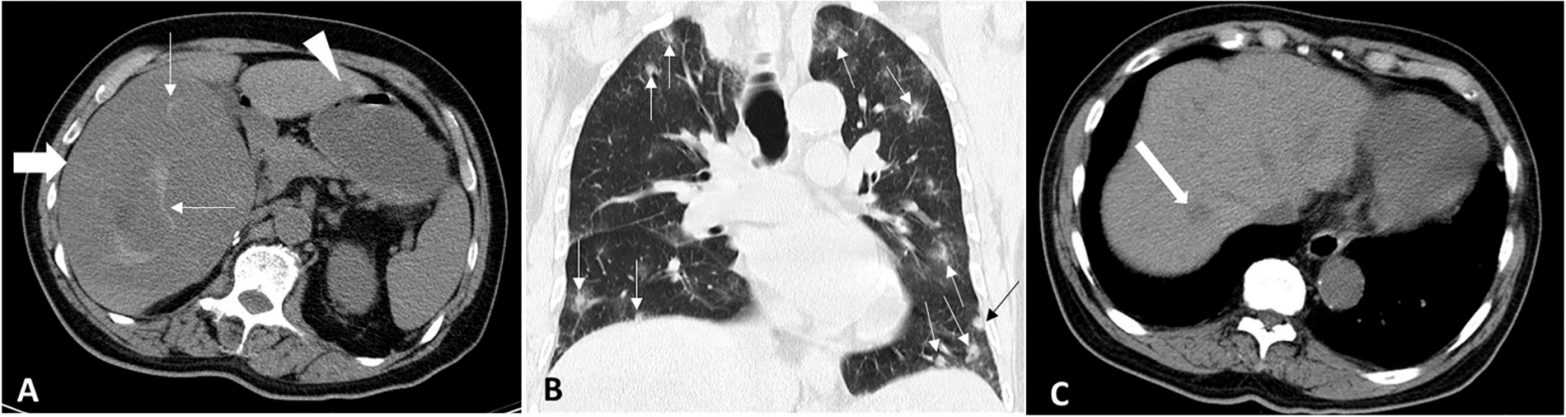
An 82-year-old man with primary hepatic angiosarcoma (case 6). (A) Transverse pre-contrast computed tomography (CT) image shows a dominant low dense mass (thick arrow) in the right lobe with small intra-tumoral high density foci (thin arrows), which suggest hemorrhage. Another smaller tumor in the left lobe of the liver is also noted (arrowhead). (B) Coronal pre-contrast CT image with lung window depicts multiple lung metastases (arrows) in the bilateral lungs. (C) Transverse pre-contrast CT image shows a small low density nodule (arrow) in the right lobe of liver. The mean value of CT Hounsfield unit (HU) for the nodule (arrow) is 39, which is greater than water attenuation (0–20 HU). This study classifies the tumor pattern of case 6 as multiple nodules with a dominant mass in liver.
